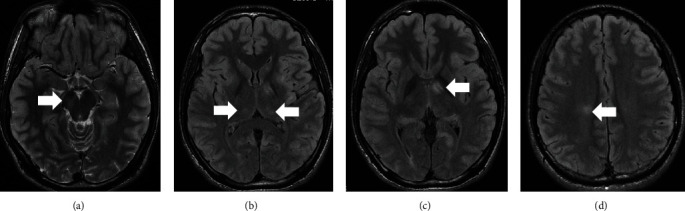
(a) T2WI imaging of the brain showed a hyperintense lesion at the right cerebral peduncle (arrow). (b) FLAIR imaging of the brain showed a hyperintense lesion at the bilateral thalami (arrow). (c) FLAIR imaging of the brain showed a hyperintense lesion at the left internal capsule (arrow). (d) FLAIR imaging of the brain showed a hyperintense lesion at the right corona radiata (arrow).
